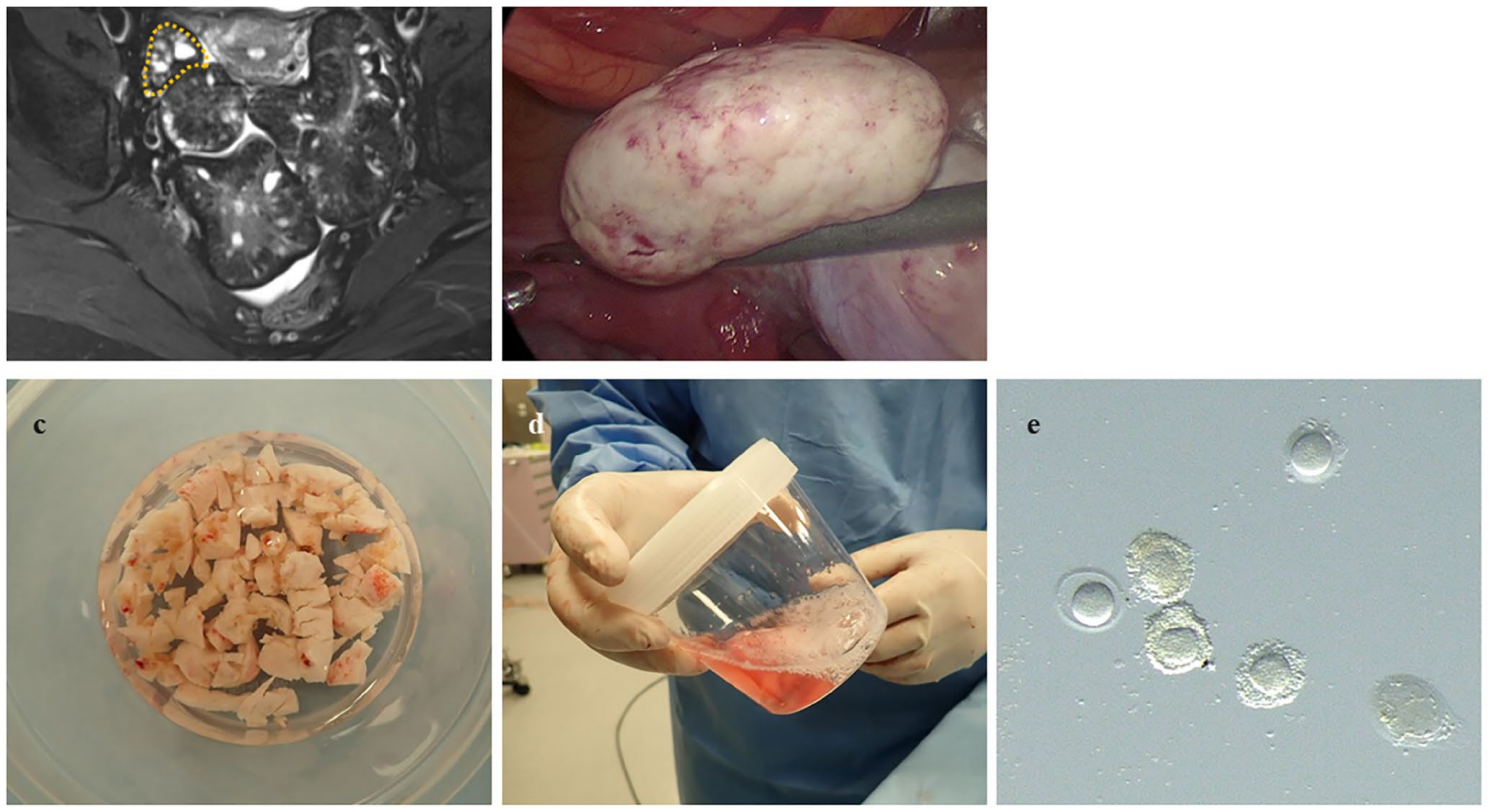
Oocyte retrieval from resected ovarian tissue. (a) Preoperative MRI (T2‐weighted image) identifying the planned resection area within the thumb‐sized (dotted line) portion of the right ovary. (b) Laparoscopic findings of the resected ovarian portion. (c) Diced ovarian tissue after resection, fragmented into 5‐mm cubic pieces. (d) Tissue pieces agitated in culture medium to facilitate oocyte retrieval. (e) Retrieved oocytes from resected ovarian tissue. Oocytes showed minimal cumulus cell attachment, characteristic of ovarian fibromatosis.
